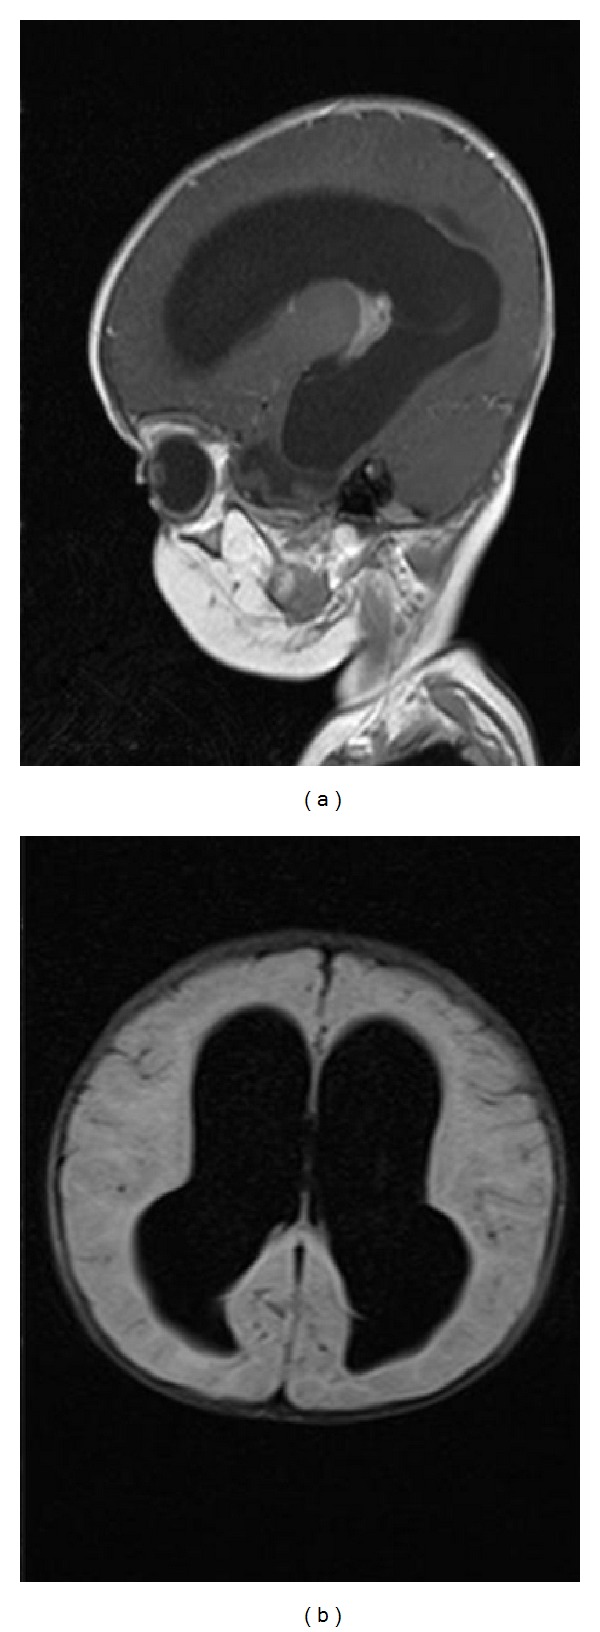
T1 sagittal (a) and T2 dark fluid transverse (b) view of brain MRI show hydrocephalus.
